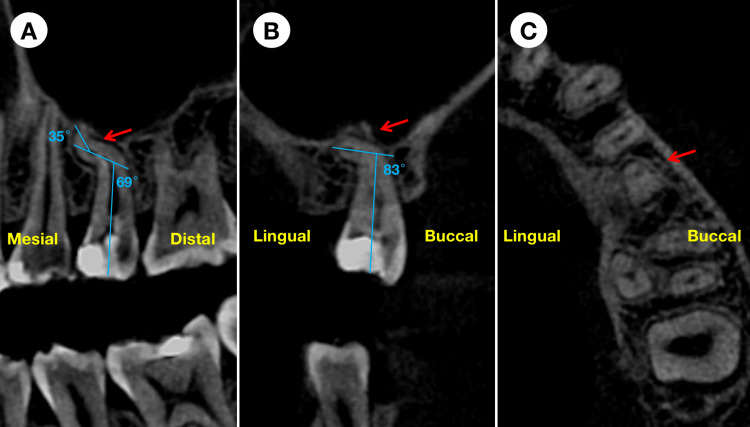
Sagittal (A), coronal (B), and axial (C) sections of cone-beam computed tomography images of the maxillary left second premolar There were multiple root curvatures, specifically mesial curvature in the sagittal section (A) and lingual curvature in the coronal section (B).
